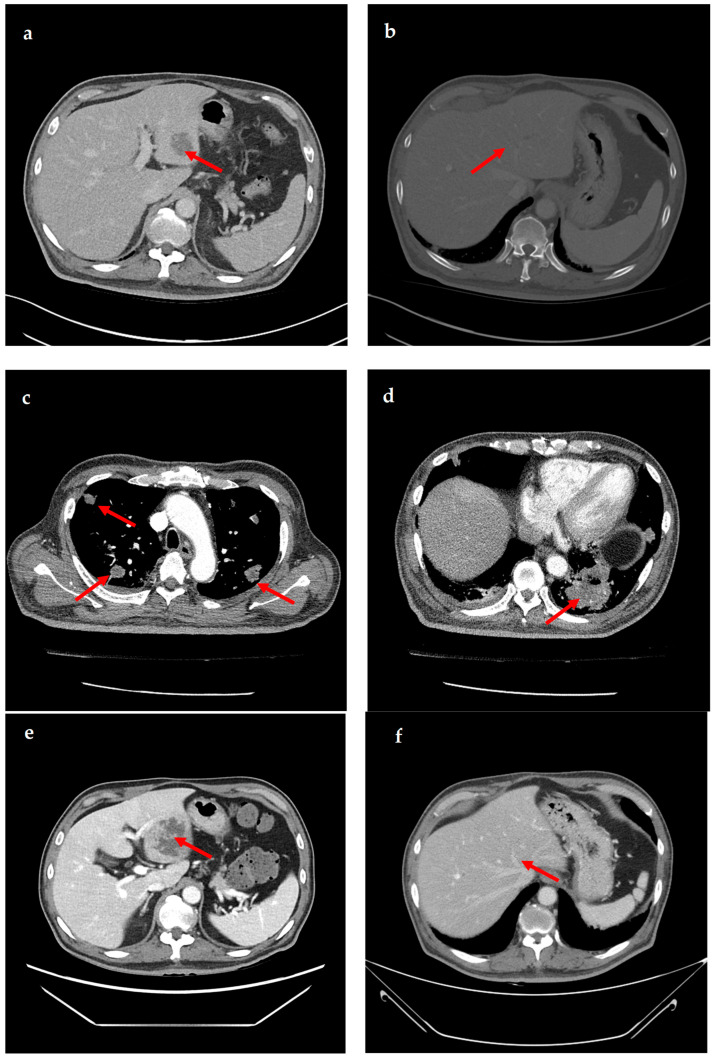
Computed tomography (CT) images showing liver abscess and septic pneumonia. (a) Gastrointestinal bleeding dynamic CT images show liver abscess (approximately 3.1 cm) at segments 2/3. (b) Gastrointestinal bleeding dynamic CT images show focal septic thromboembolism in the left hepatic vein. (c,d) Chest CT images show multiple mass-like consolidations in both lungs. (e) Abdominopelvic CT images show an increased liver abscess (approximately 6.9 cm) at segments 2/3. (f) Abdominopelvic CT images show that the liver abscess has resolved.
